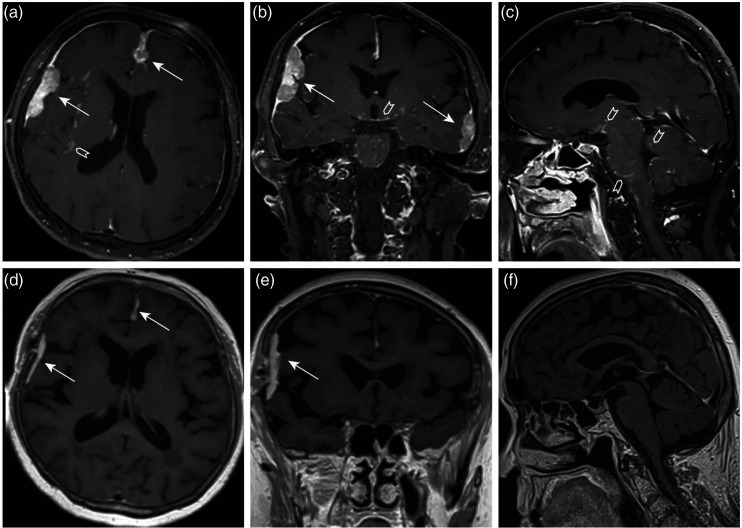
The pretreatment magnetic resonance (MR) images are presented in the upper row (A)–(C). Axial, coronal, and sagittal postgadolinium T1-weighted sequences show multiple dural lesions (white arrows) and subtle leptomeningeal contrast enhancement (white arrowheads) consistent with neurosarcoidosis. The follow-up MR images performed 2 months after the initiation of corticosteroid therapy show a good treatment response in dural lesions (D, E, white arrows) and complete resolution of the leptomeningeal enhancement (D)–(F).
